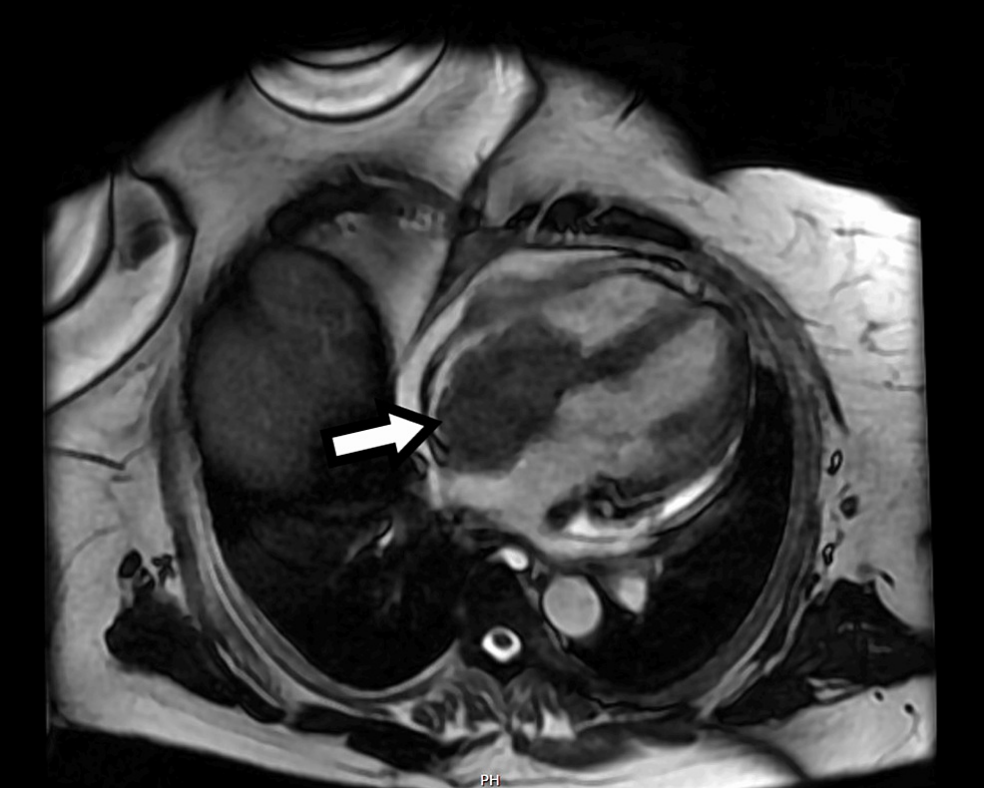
MRI showing the mass in the right atrium
